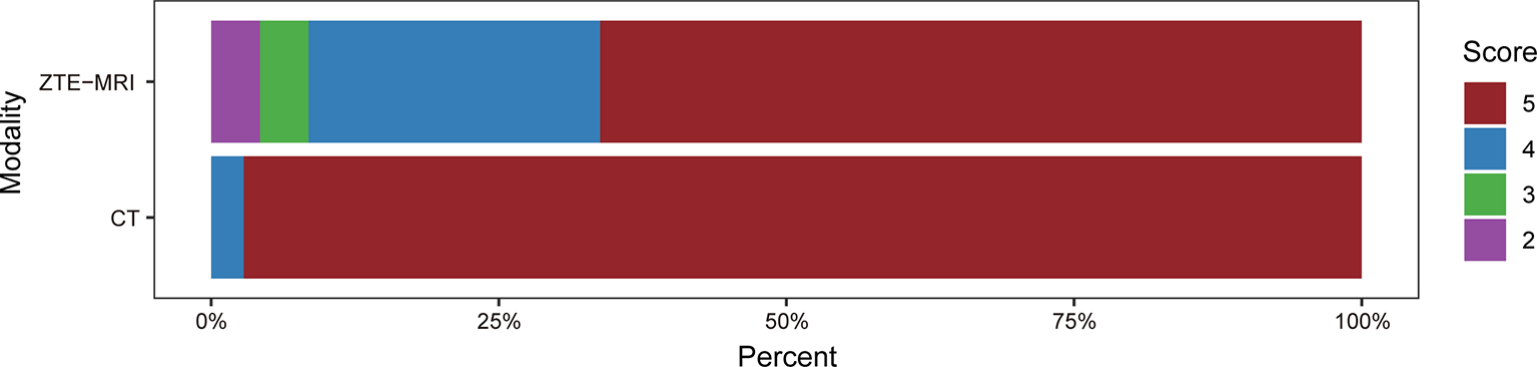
Histogram of the subjective image quality scores for lung ZTE-MRI and CT.
